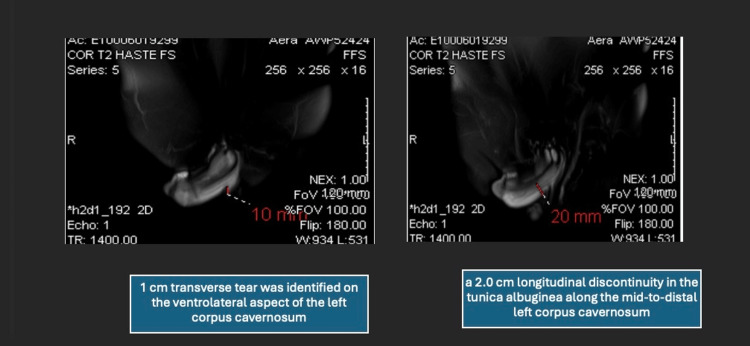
T2-weighted MRI of penile fracture. Axial and sagittal T2-weighted MRI images reveal a 2.0 cm longitudinal discontinuity (right panel) in the tunica albuginea of the mid-to-distal left corpus cavernosum. A contained hematoma measuring 2.0 cm is seen overlying the defect, confined by Buck’s fascia. Urethra is mildly compressed but intact. No contralateral involvement is observed. MRI, magnetic resonance imaging.
